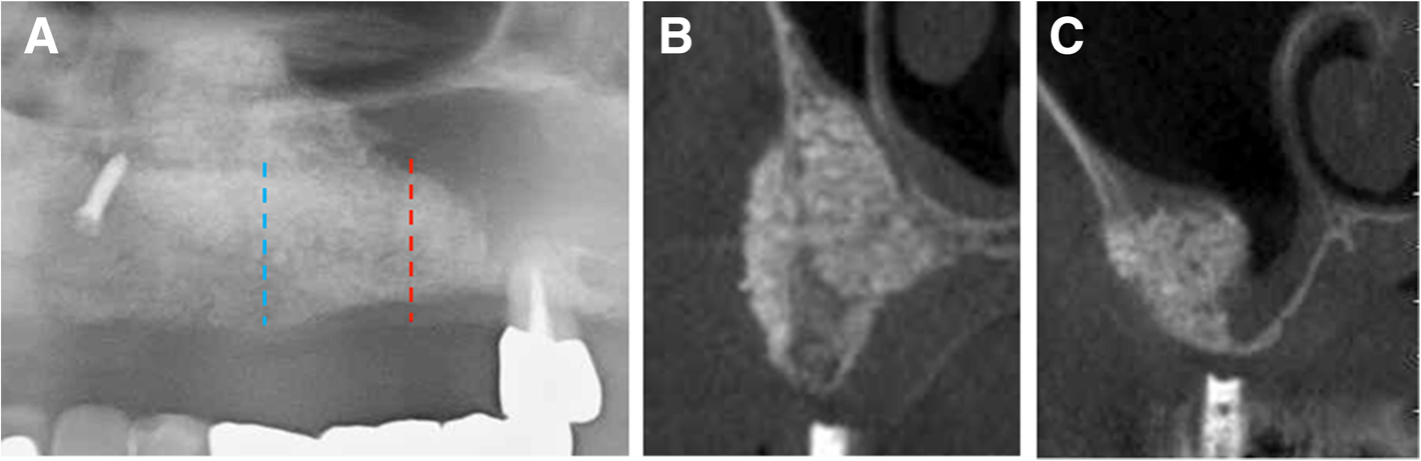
Radiographic image, 5 months after sinus bone augmentation. a Orthopantomography shows inadequate bone height observed in sites #26 (*red line*). b Coronal CT image of the *blue line*; vertical bone height is 15 mm. Horizontal augmentation is also achieved. c Coronal CT image of the *red line*; no bone augmentation was achieved in the palatal aspect of sinus cavity
